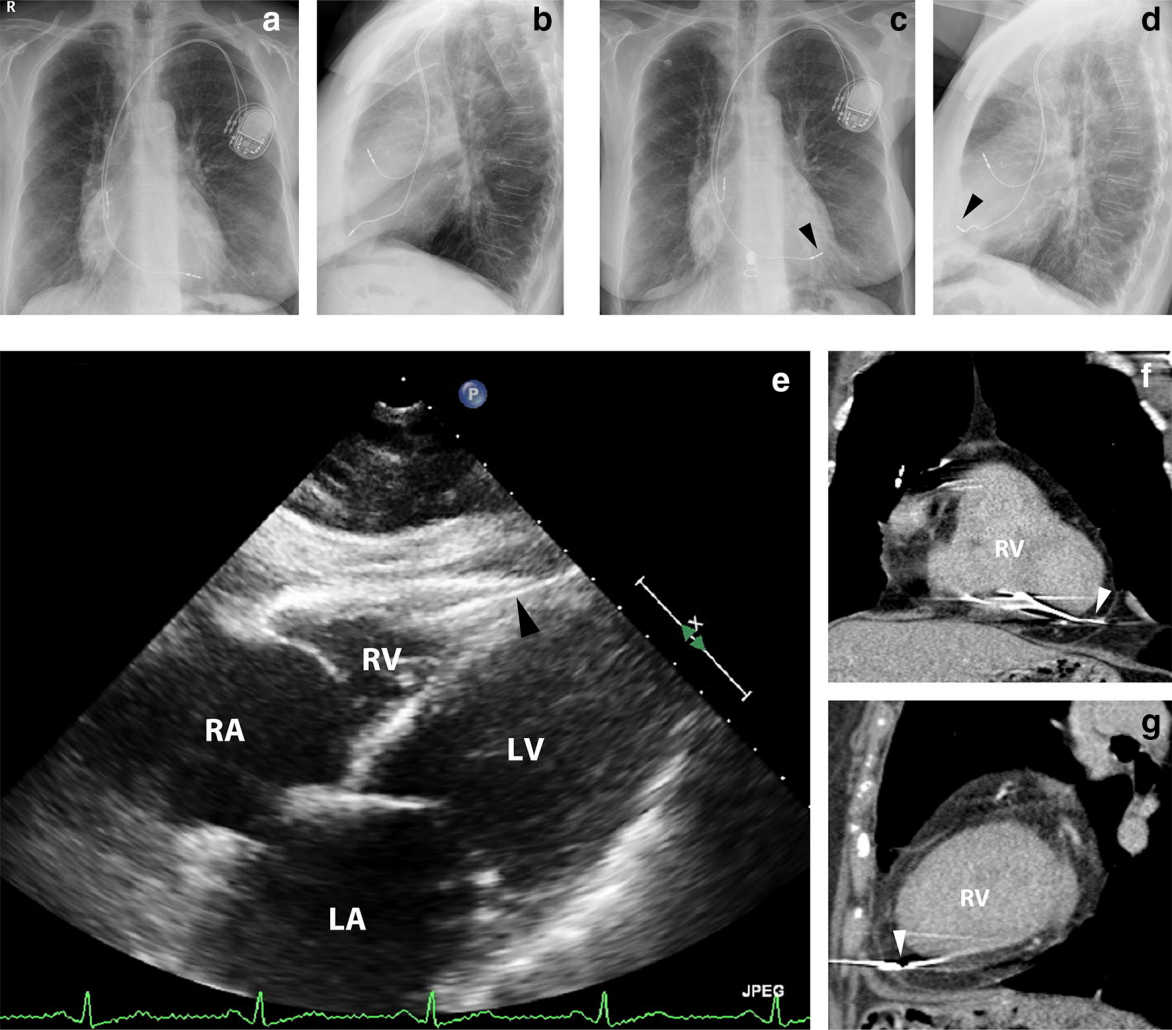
a Chest radiography [postero-anterior (PA) view] the day after pacemaker implantation. b Lateral view. c Chest radiography (PA view) upon presentation to the emergency room showing an altered position of the RV lead (*arrowhead*). d Lateral view (*arrowhead* shows altered lead position). e Transthoracic echocardiography (subcostal view) suggesting myocardial perforation of the RV lead through the RV apex (*arrowhead*), but without pericardial effusion, raising doubt as to whether the lead tip lay in the pericardial space. See also online video. *LA* left atrium, *LV* left ventricle, *RA* right atrium, *RV* right ventricle. f Thoracic computed tomography (coronal view) with lead tip clearly visible through the myocardium (*arrowhead*). *RV* right ventricle. g Thoracic computed tomography (sagittal view) showing lead tip running through myocardium (*arrowhead*). *RV* right ventricle
